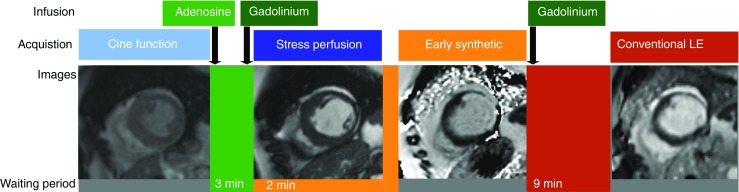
Cardiac MR imaging protocol. Cine function imaging is followed by injection of adenosine. After a waiting period of 3 min acquisition of the first-pass perfusion of gadolinium contrast is achieved. Two min after the injection of the first bolus of gadolinium, early synthetic inversion recovery (IR) images are acquired. After this, a second bolus of gadolinium is injected and after an additional waiting period of 9 min the conventional late gadolinium enhancement (LGE) IR images are acquired
